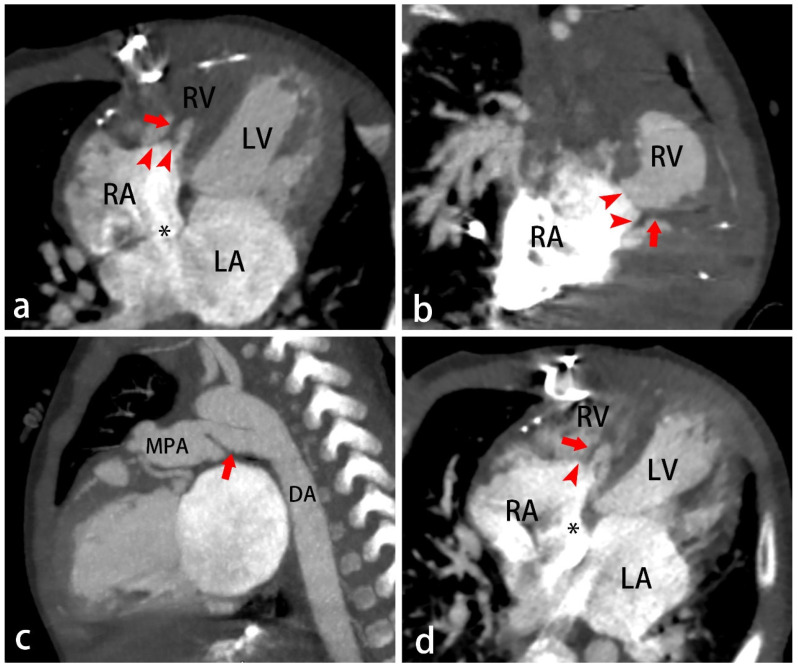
A 30-day-old boy with tricuspid valve dysplasia. CT angiography four-chamber view (a) and two-chamber view (b) show thickening of the tricuspid valve leaflets ((a,b), red arrowheads), which are connected to the thickened papillary muscle ((a,b), red arrows). The right ventricle is significantly hypoplastic and an atrial septal defect was also seen ((a,d), black asterisk). Maximum intensity projection (MIP) image shows the presence of patent ductus arteriosus (PDA) ((c), red arrow). CT angiography diastolic four-chamber view still shows restricted opening of the tricuspid valve leaflets (red arrowhead in (d)) and thickened papillary muscle ((d), red arrow). LV, left ventricle; LA, left atrium; RA, right atrium; RV, right ventricle; MPA, main pulmonary artery; DA, descending aorta.
